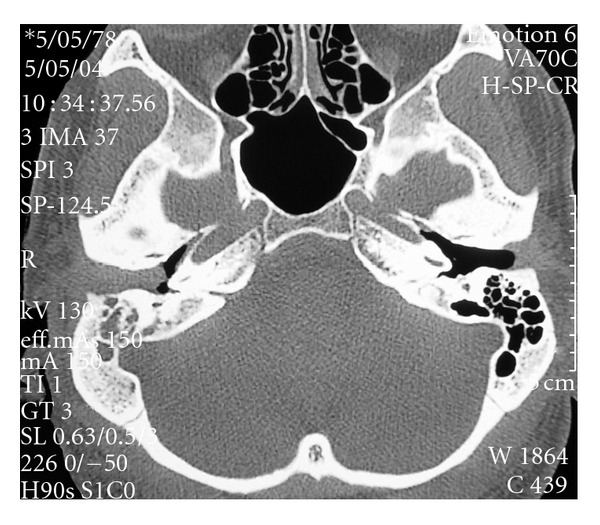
Axial CT of both temporal bones. The mastoid air cells on the right appear filled with a soft tissue density as well as the external auditory canal. The middle ear is filled with air.
